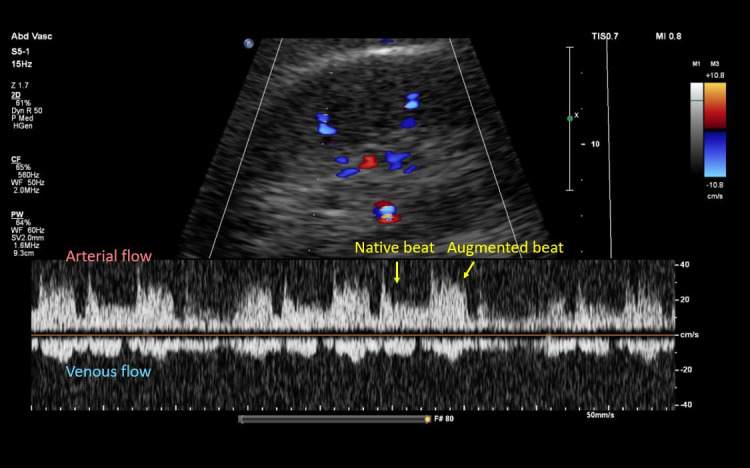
Intrarenal Doppler at intra-aortic balloon pump (IABP) frequency of 2:1.
